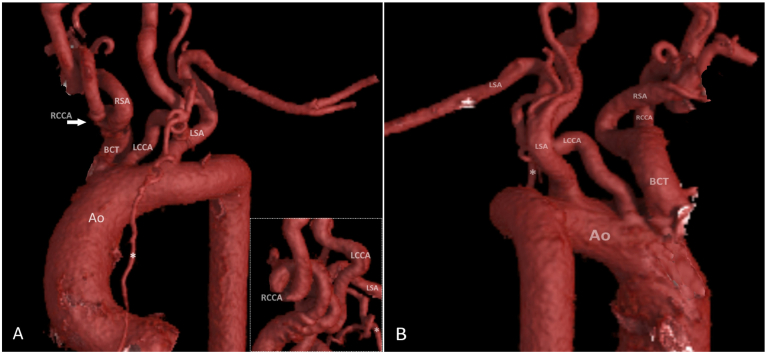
Supra-Aortic Trunk Tortuosity Demonstrating Systemic Vascular Involvement Three-dimensional CT angiographic reconstruction of the aortic arch and supra-aortic branches. (A) Left oblique-coronal view showing marked tortuosity of the right common carotid artery (RCCA) (dotted square). (B) Posterior oblique view demonstrating looping of both carotid and subclavian arteries from their origin at the aortic arch. These findings confirm diffuse systemic arterial involvement consistent with arterial tortuosity syndrome. Ao: Aorta; BCT: Brachiocephalic trunk; SVC: Superior cava vein; RCCA: Right common carotid artery; LCCA: Left common carotid artery; RSA: Right subclavian artery; RSV: Right subclavian vein ; ∗: Internal thoracic artery; LPA: Left pulmonary artery.
